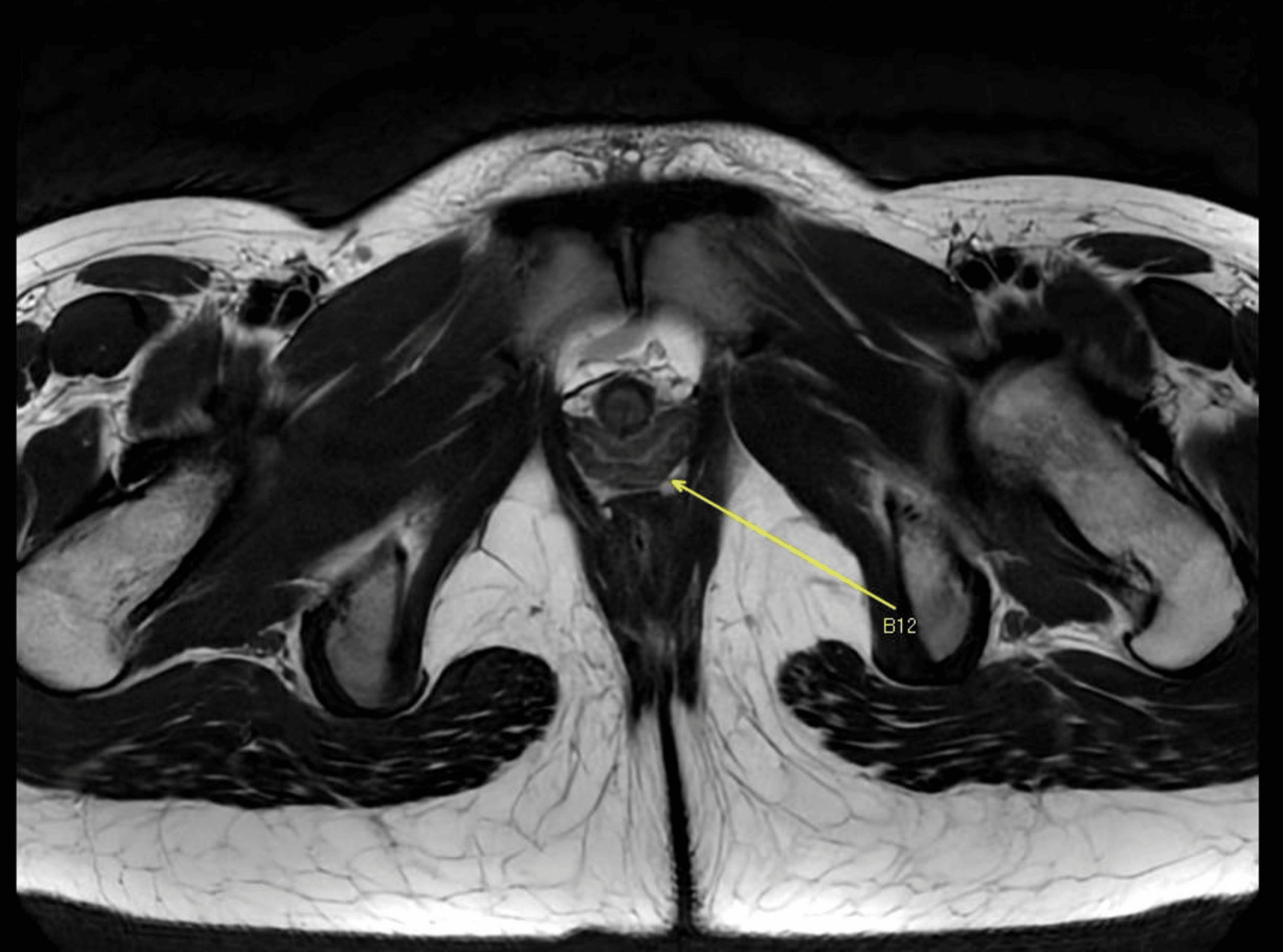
Axial view on MRI showing uterine didelphys and a common vaginal canal MRI: magnetic resonance imaging
